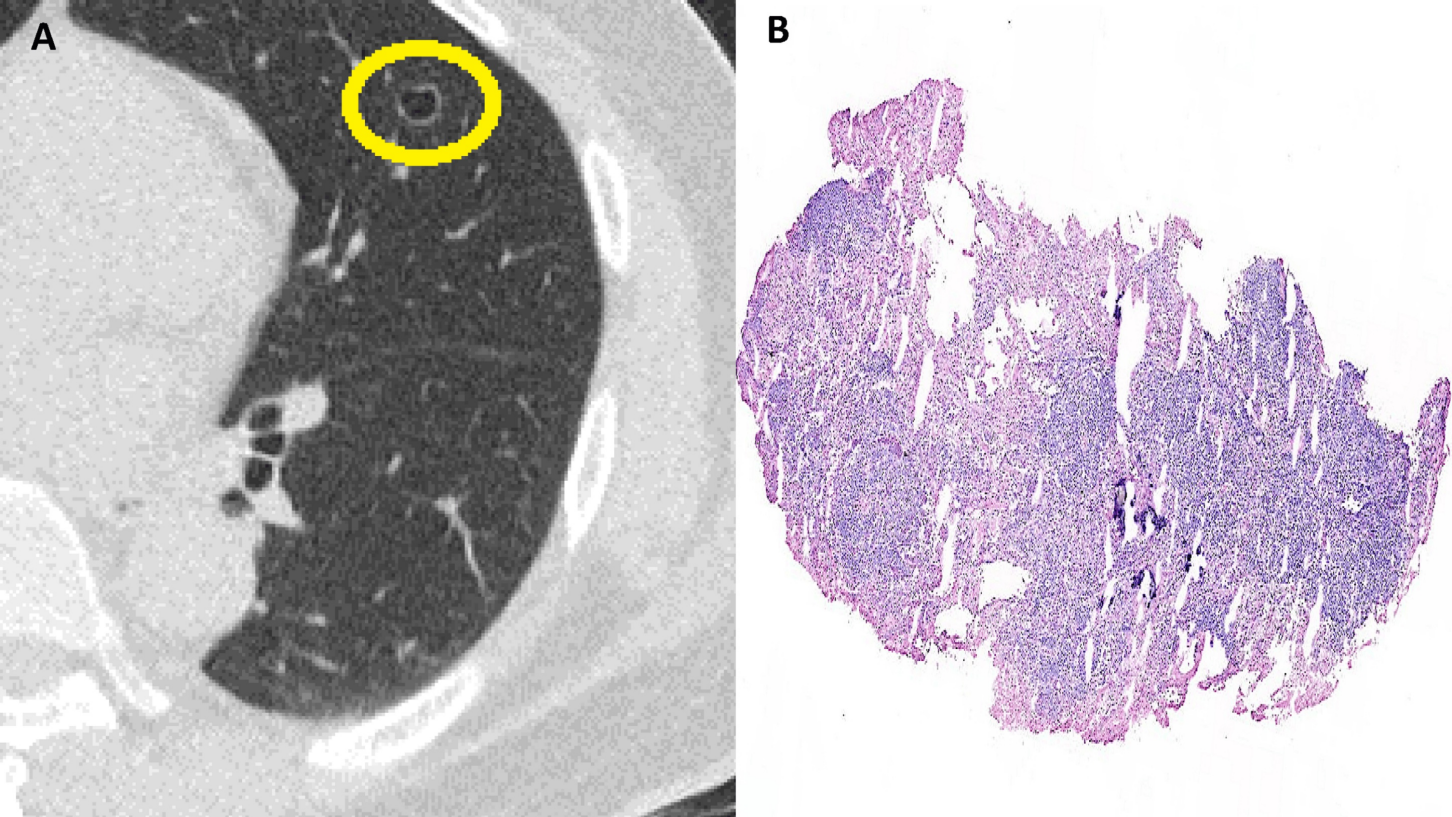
Chest image and intraoperative frozen section (A) CT of the chest showing a cystic lesion at LUL (circle). (B) Intraoperative frozen section revealing a round lesion rich in lymphocytes (original magnification, ×20) CT: computed tomography; LUL: left upper lobe
